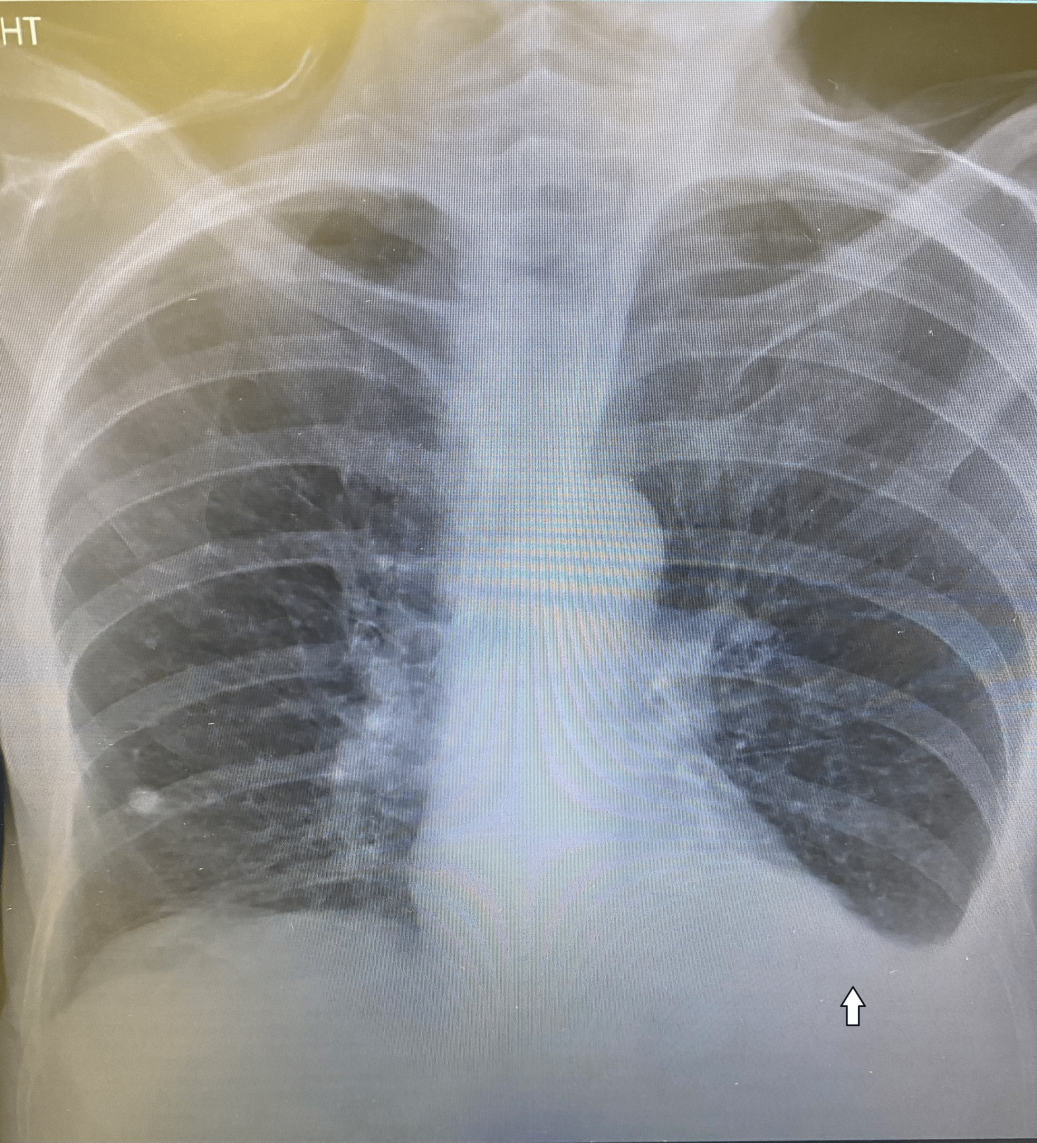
Anteroposterior chest X-ray 19 days before arrival at the emergency department.
